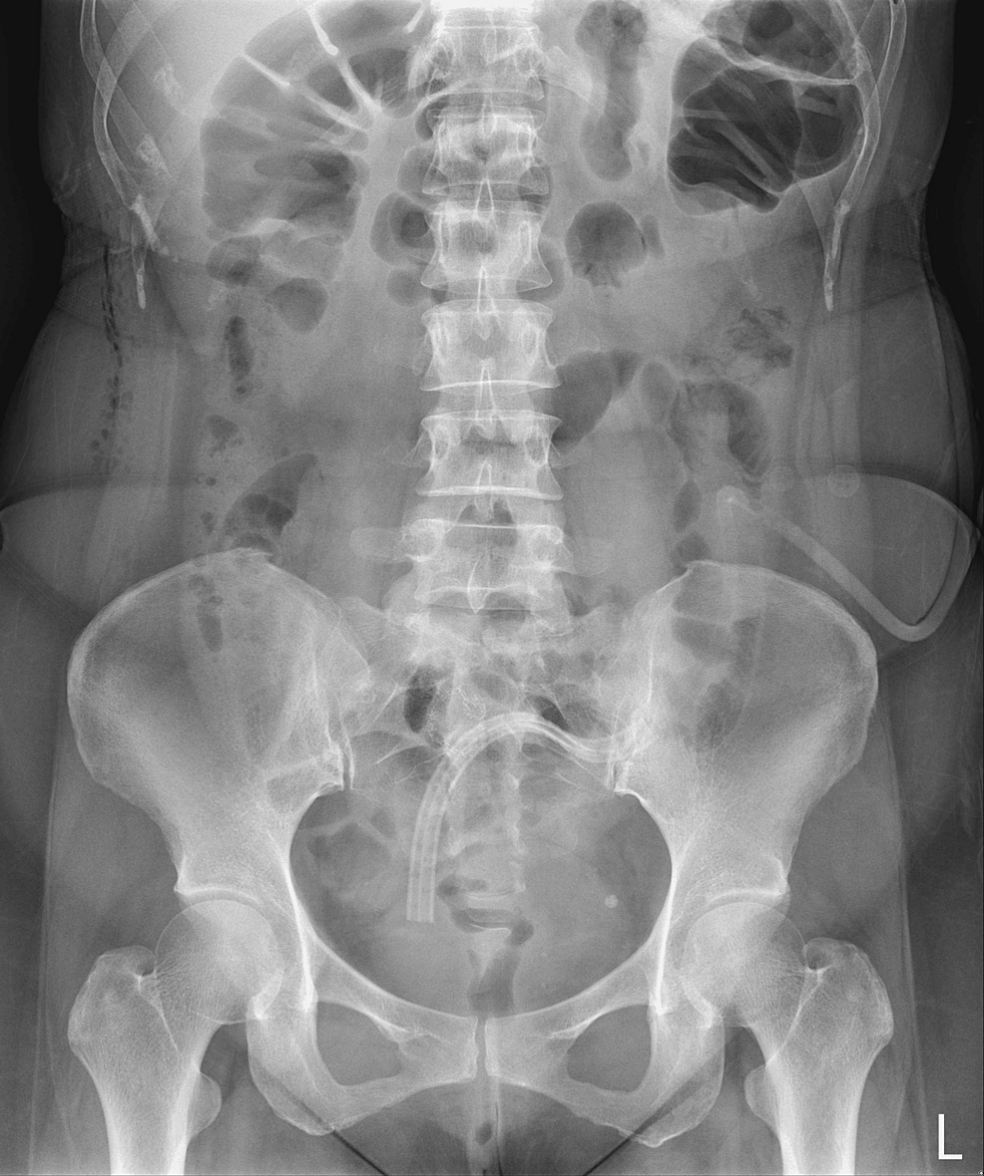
Post-procedure abdominal X-ray. The abdominal X-ray after the sigmoidoscope showed much improvement in colon gas, with the gas passing through anastomosis to the rectum.
